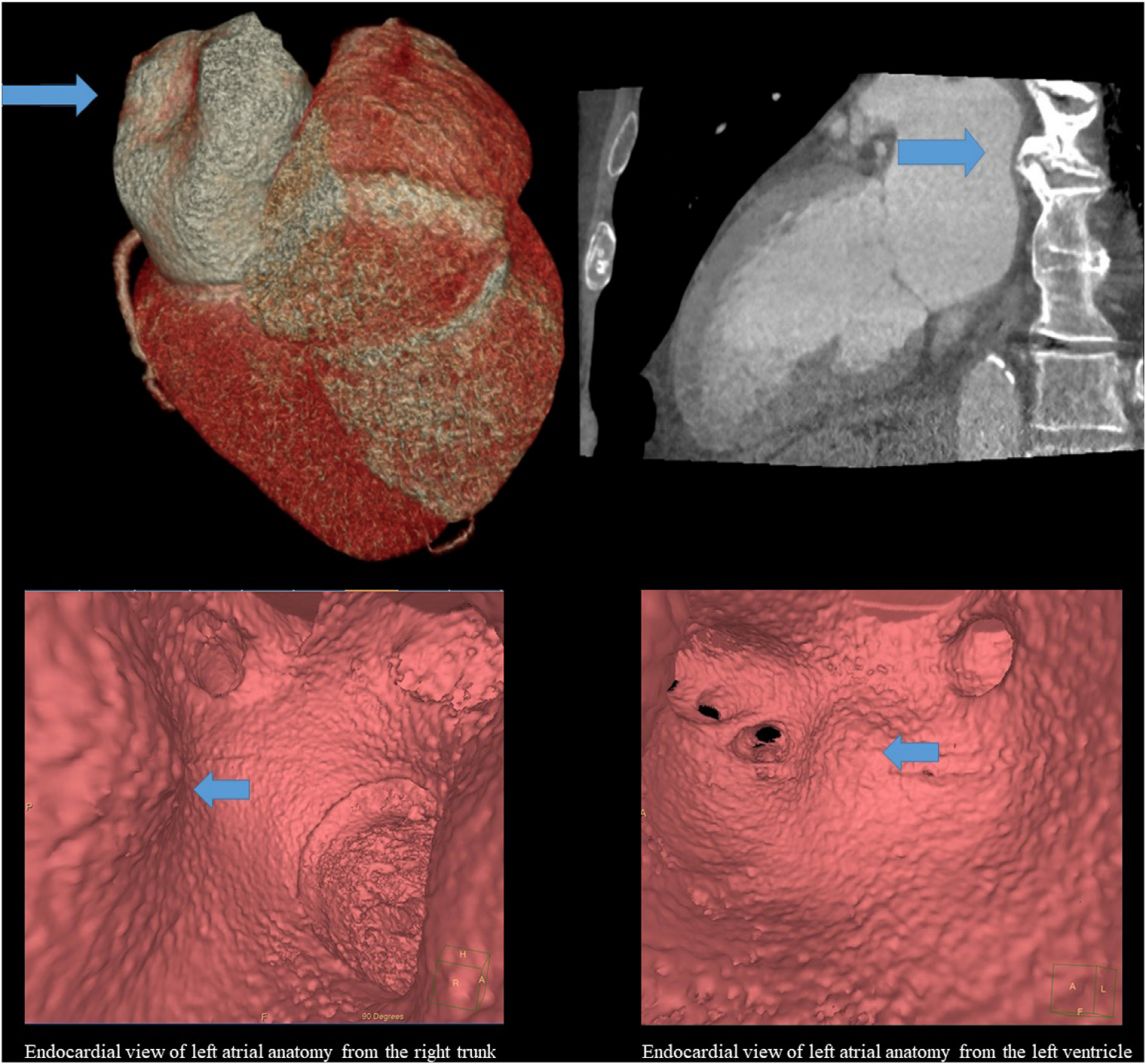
Cardiac computed tomography angiography showing compression on the posterior wall of left atrium caused by vertebral osteophyte (A: 3D volumetric rendering of the heart, posterior view and 2D view of the left atrium and ventricle). The blue arrows indicate the site of compression on the posterior wall of left atrium caused by vertebral osteophyte as depicted on the endocardial views from the right common trunk and from the left ventricle (B).
